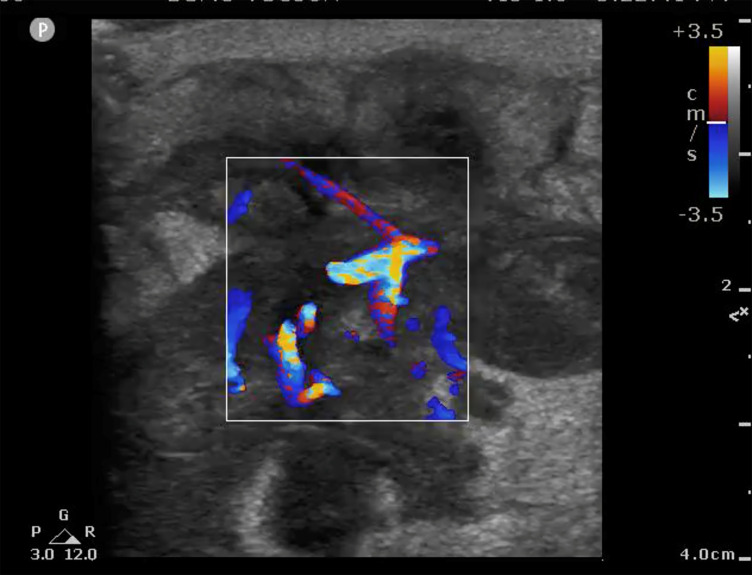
Irregular, highly vascularized structure found on a breast point-of-care ultrasound examination concerning for malignancy, later confirmed to be invasive ductal carcinoma.
